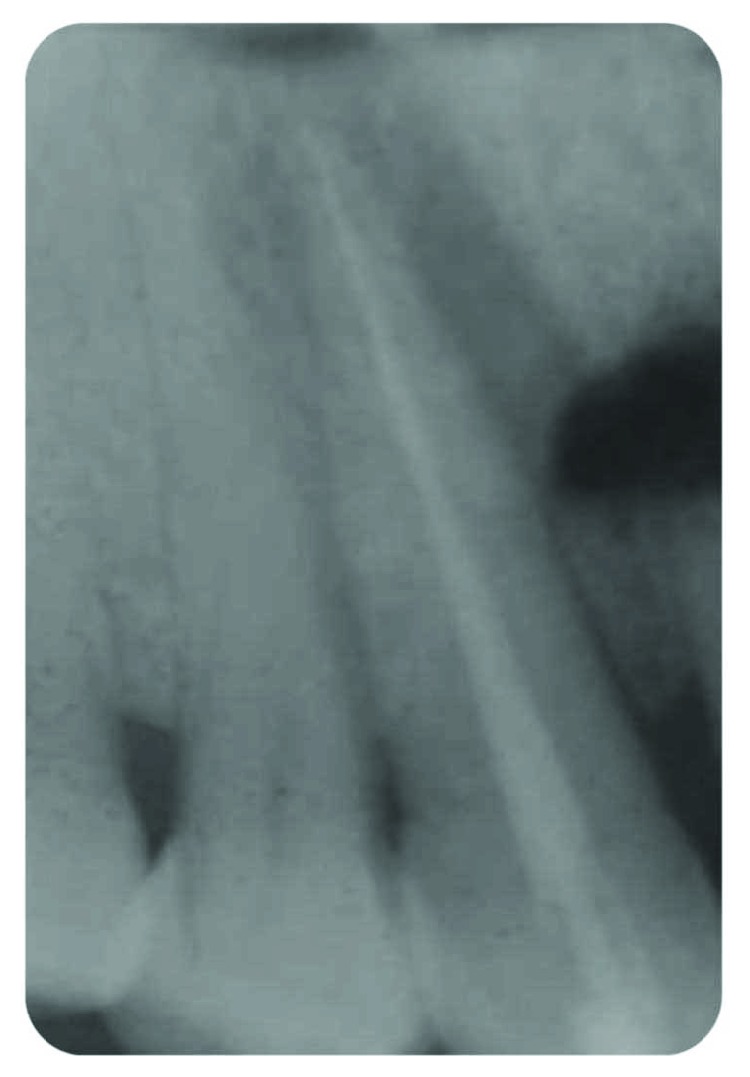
Two months' recall radiograph showing a continuous bone reorganization.
